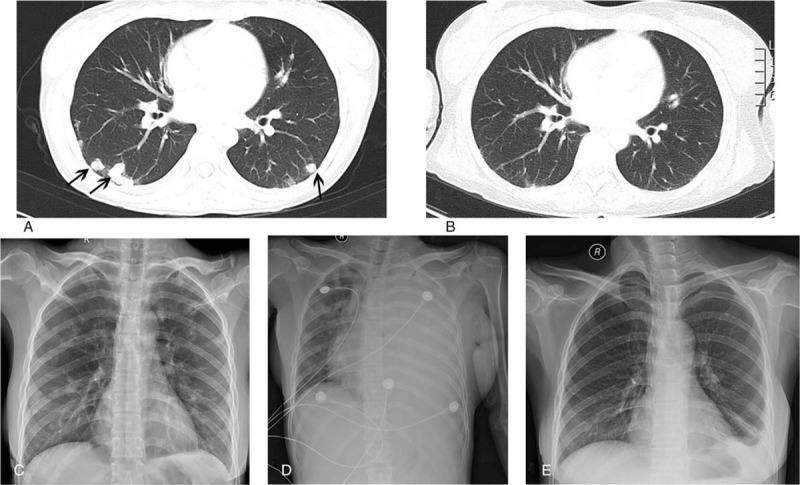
Lung computed tomography (CT) images and chest radiographs of the patient at different stages of treatment. (A) Multiple nodules of bilateral pulmonary were shown on CT image before chemotherapy (black arrows, metastatic nodules). (B) CT image revealed an almost normal lung field after treatment. (C) Multiple lung nodules were seen in bilateral lung field before treatment. (D) Large-scale pleural effusion in left lung field indicated massive hemorrhage of lung metastasis on the first day of the second chemotherapy. (E) Only little pleural effusion was in left lung field after treatment.
